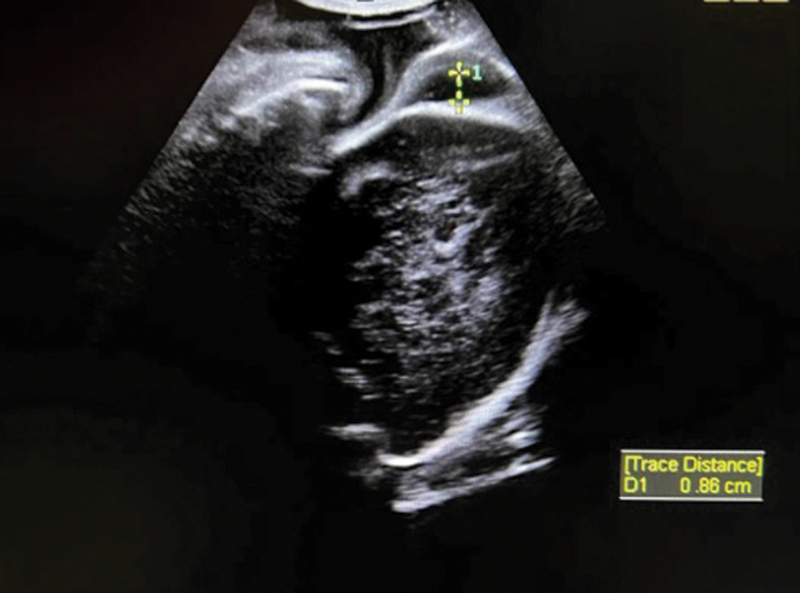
Ultrasound image in the delivery room showing the measurement of caput succedaneum.
